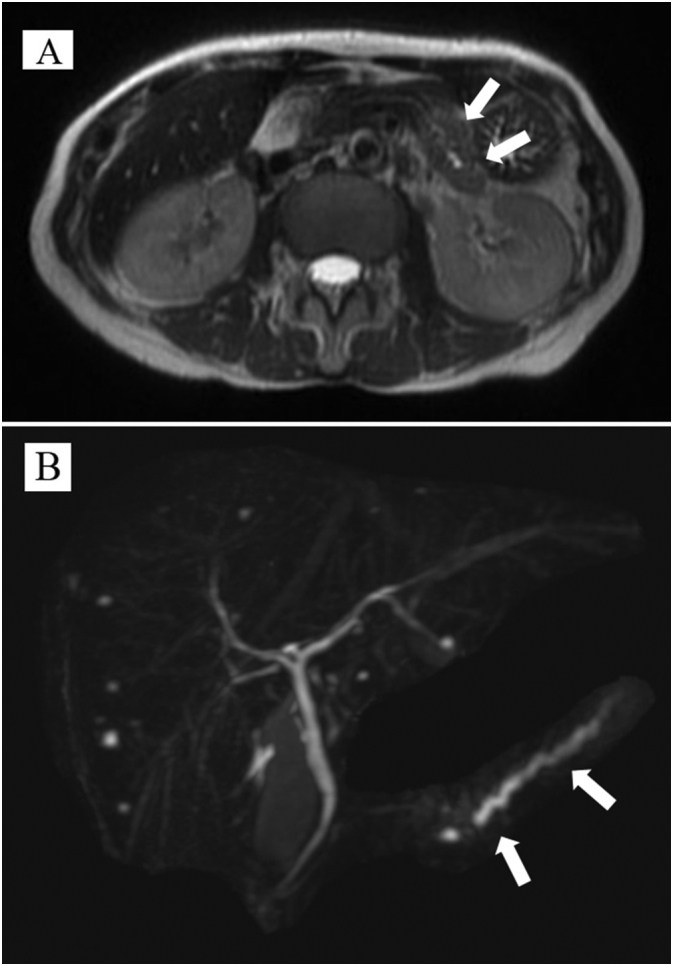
MRI scan findings. A: A tumor showing faint, high signal intensity (white arrow) on a T2-weighted image. B: The main pancreatic duct is dilated (white arrow) secondary to the tumor obstruction. MRI: magnetic resonance imaging.
